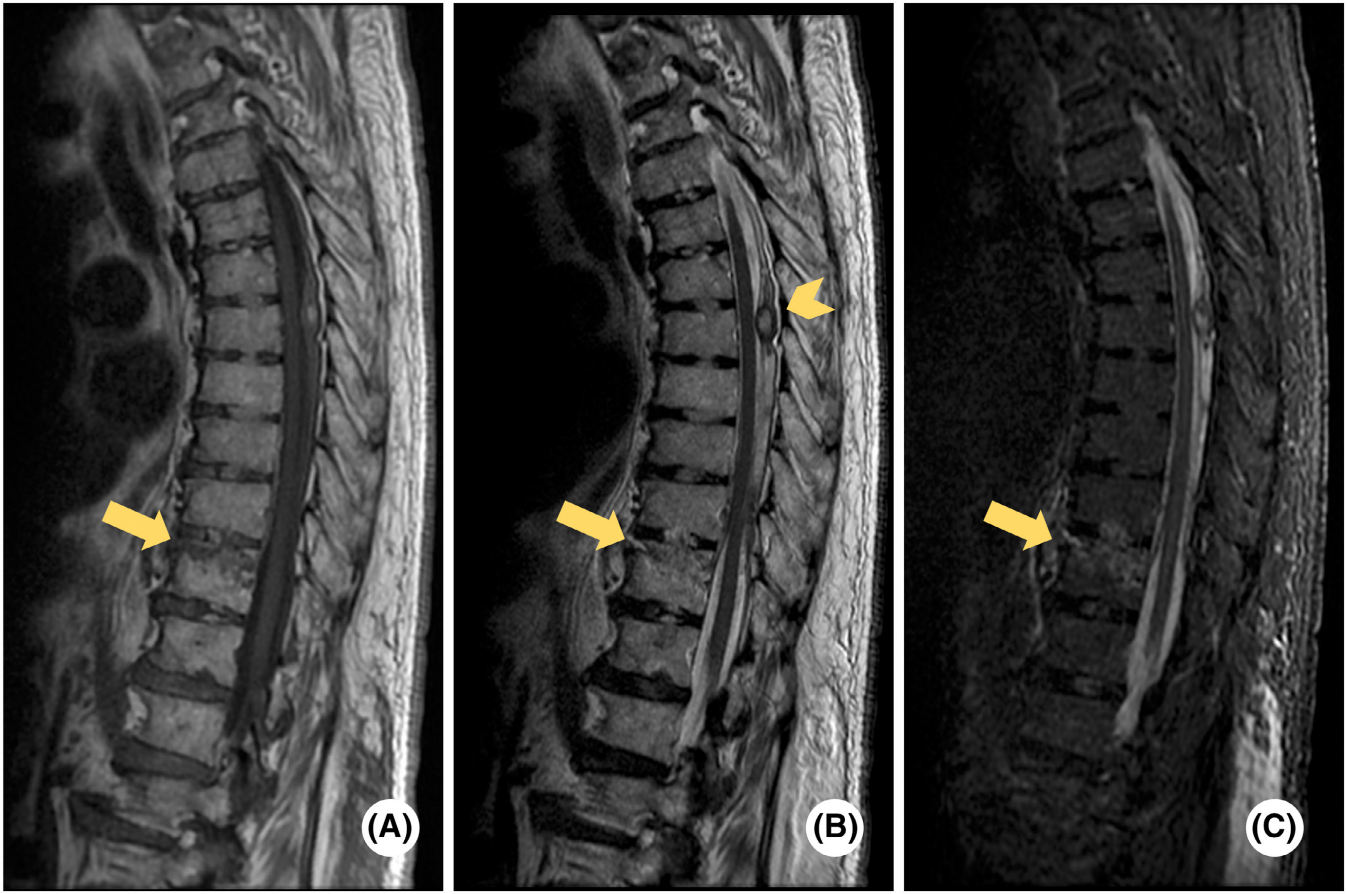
Spinal magnetic resonance imaging (MRI) on Day 3. Thoracic vertebral fracture (yellow arrows) shown in (A–C) of spinal MRI. Thoracic epidural hematoma indicated by yellow arrowhead in B.
